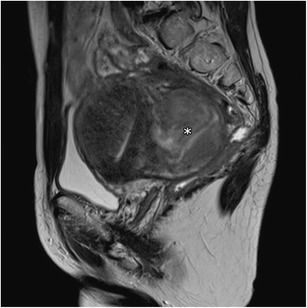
Leiomyoma: Sagittal T2-weighted image; heterogeneous and hypointense mass with well-defined borders, with mass effect on adjacent tissues (asterisk) representing leiomyoma. There are also features suggestive of adenomyosis
